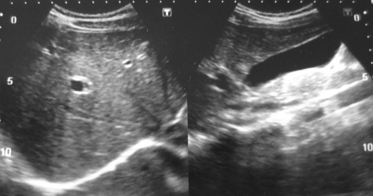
Ultrasound scan of splenic microabcesses.
